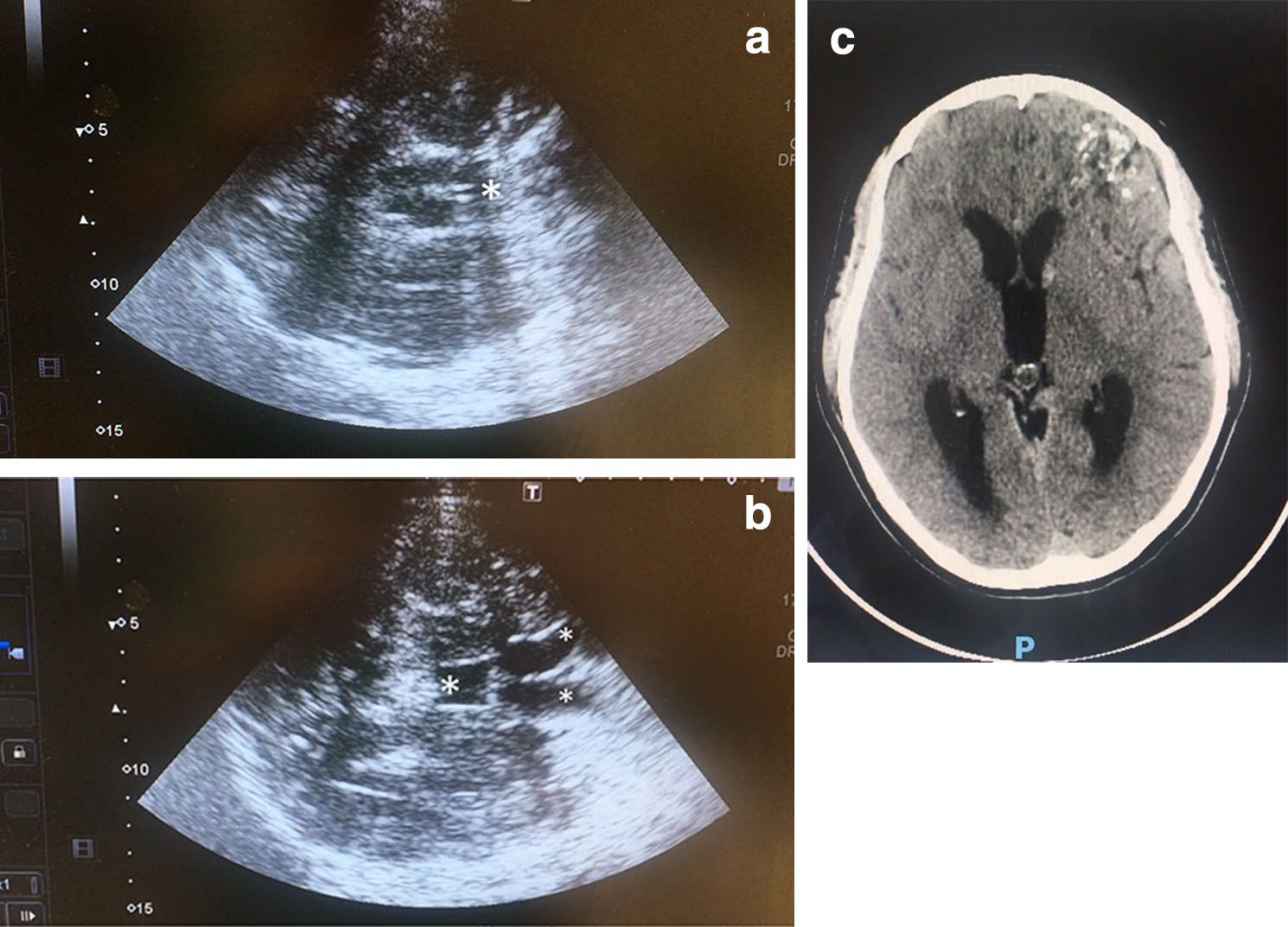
In a mesencephalic plane (a), two central pulsating hyperechoic lines, cerebral aqueduct (asterisk), were wrongly considered as third ventricle. Tilting the probe 10° cranially, in a diencephalic plane (b), third ventricle(large asterisk) and frontal horns of lateral ventricles (small asterisks) were observed, and hydrocephalus was diagnosed. A CT scan (c, EMS) confirmed the diagnosis
